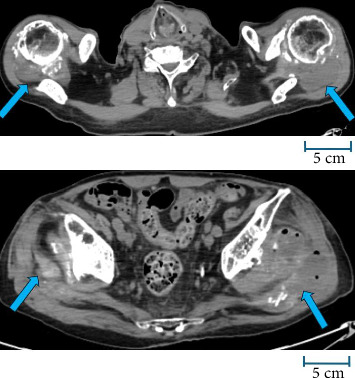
Simple CT scan of the trunk. The blue arrow indicates abscess formation. The scale bar represents a length of 5 cm to reflect the correct physical dimensions.
